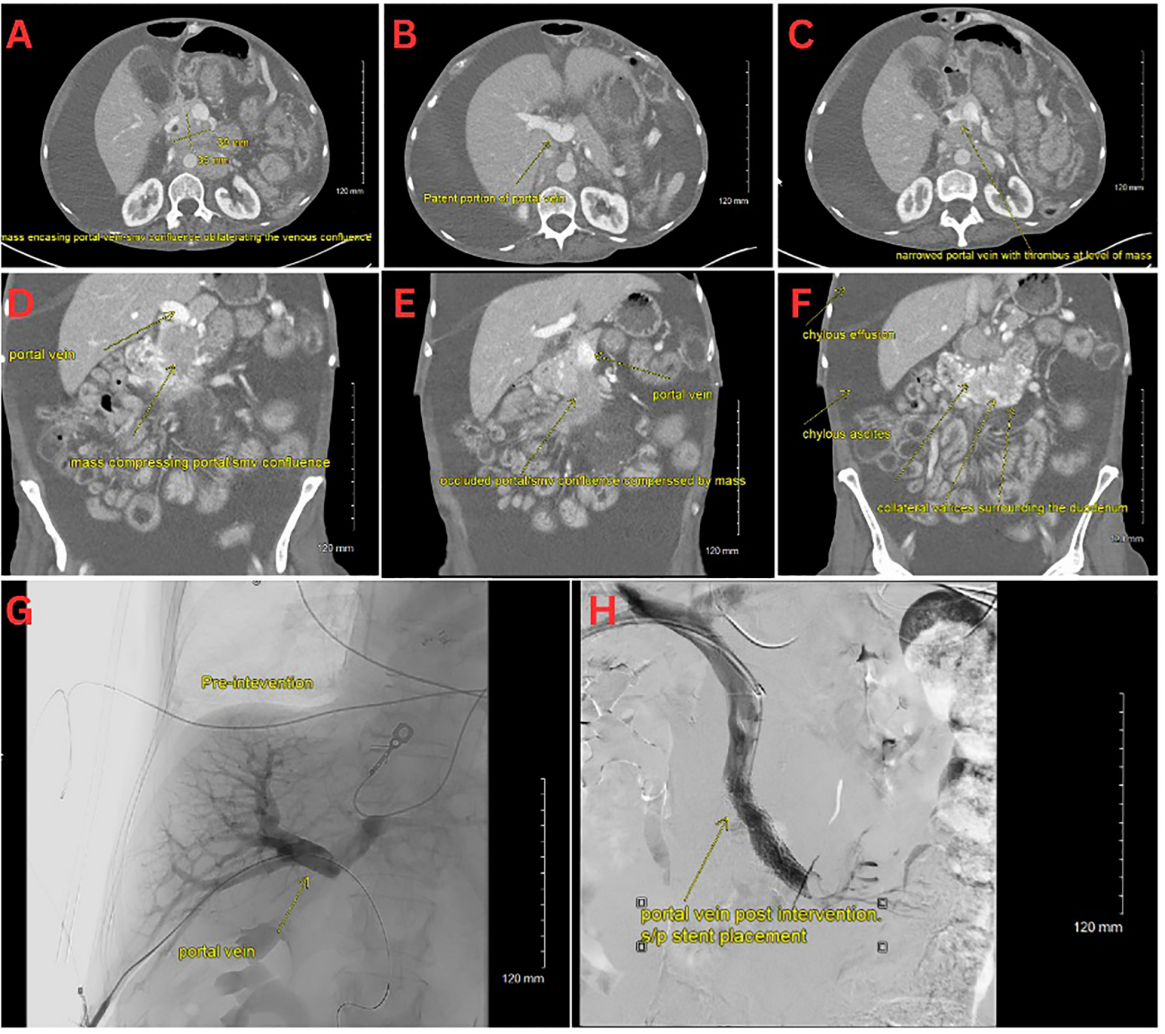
(A–C) CT scan with an axial view of the pNET mass. (D–F) CT scan with a coronal view of the pNET mass. (G) Venogram showing portal vein prior to stent deployment with no distal contrast visualized. (H) Venogram post SMV stent deployment with subsequent patency.
